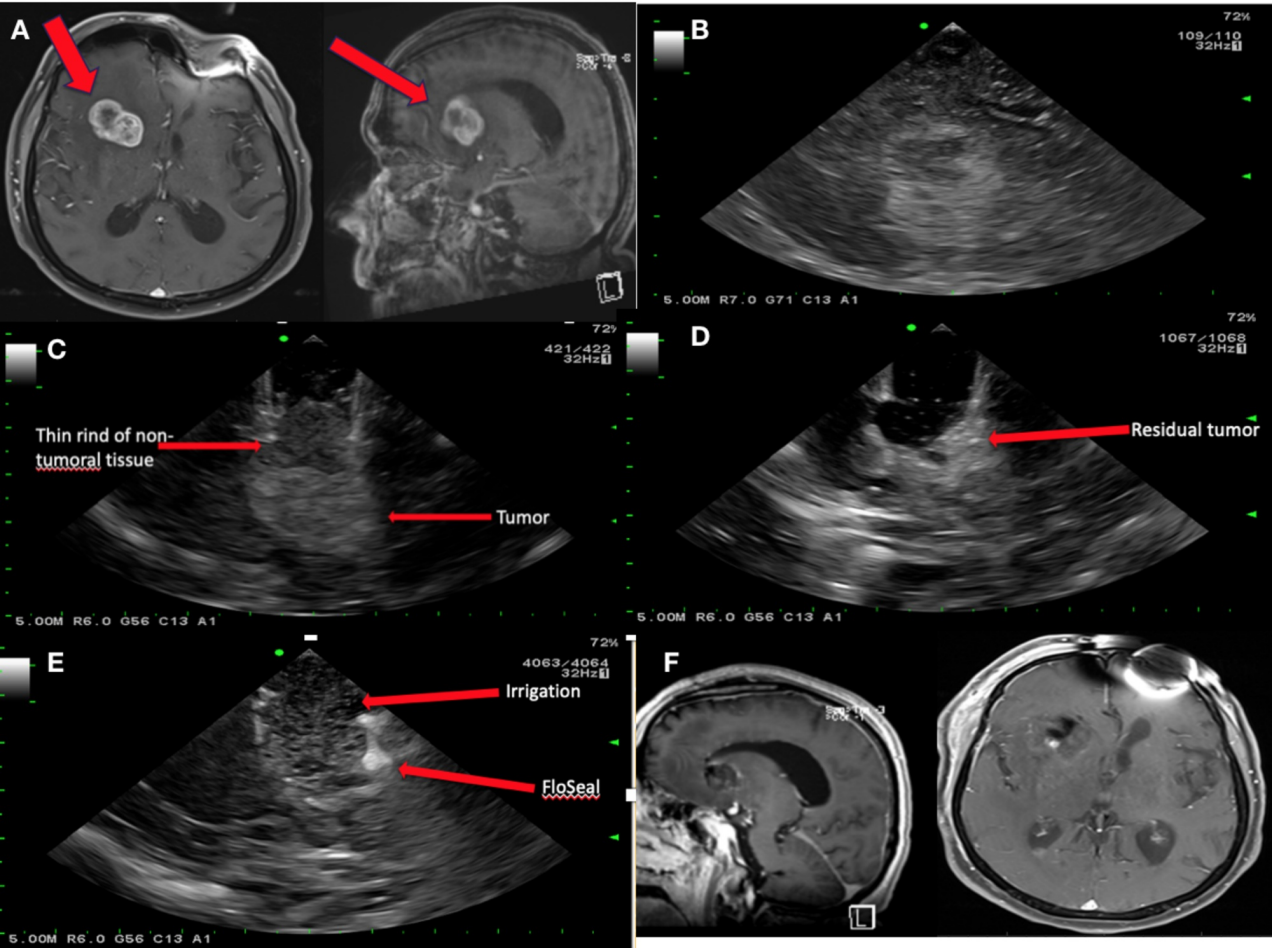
Magnetic resonance imaging (MRI) and ultrasound pre- and post-resection using BrainPath. (A) A T1-weighted MRI with contrast (axial sequence on the left and sagittal sequence on the right) showing a right periventricular enhancing lesion. (B) The use of intraoperative ultrasound after the craniotomy is performed to identify the tumor. (C) The use of intraoperative ultrasound to visualize the thin rind of non-tumoral tissue that can obscure the tumor as well as the tumor which is located deep to that rind. (D) The use of intraoperative ultrasound to show gross residual tumor. (E) The use of intraoperative ultrasound to show gross total resection, the irrigated surgical cavity as well as the applied hemostatic matrix. (F) A T1-weighted postoperative MRI with contrast (sagittal sequence on the left and axial sequence on the right) showing no residual tumor.
